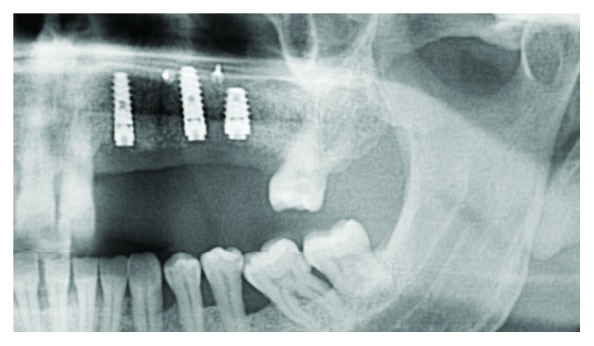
Orthopantomography after dental implant surgery.
